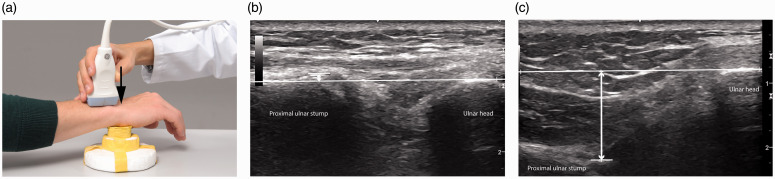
Sonography to assess the stability of the DRUJ of the operated hand (a).
